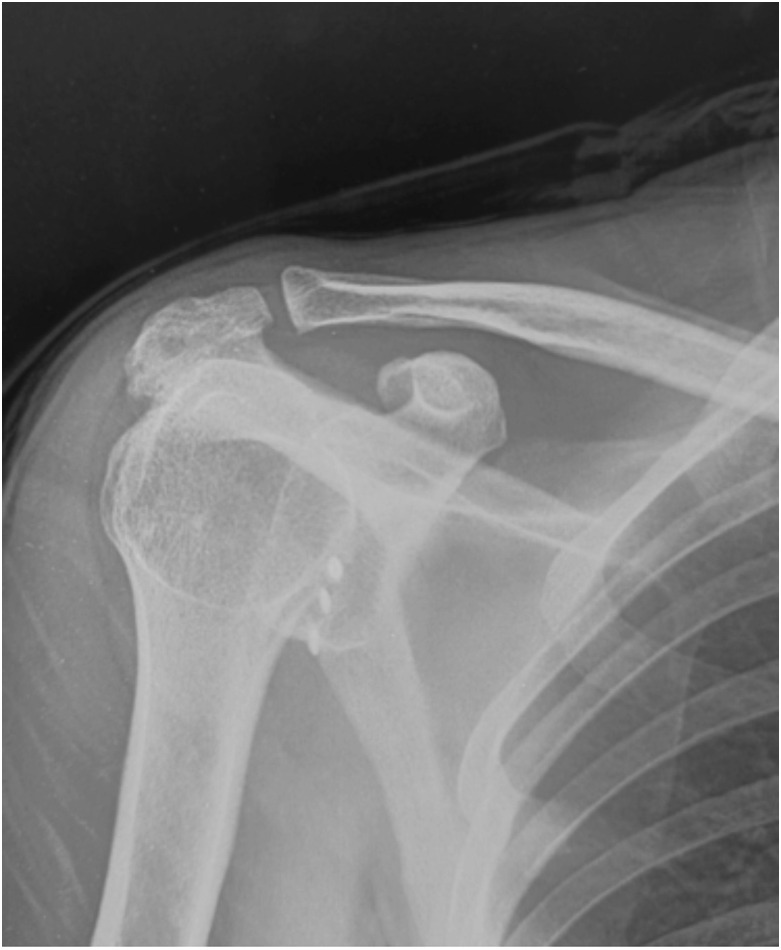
Radiograph image of Bankart repair using metal anchor.
